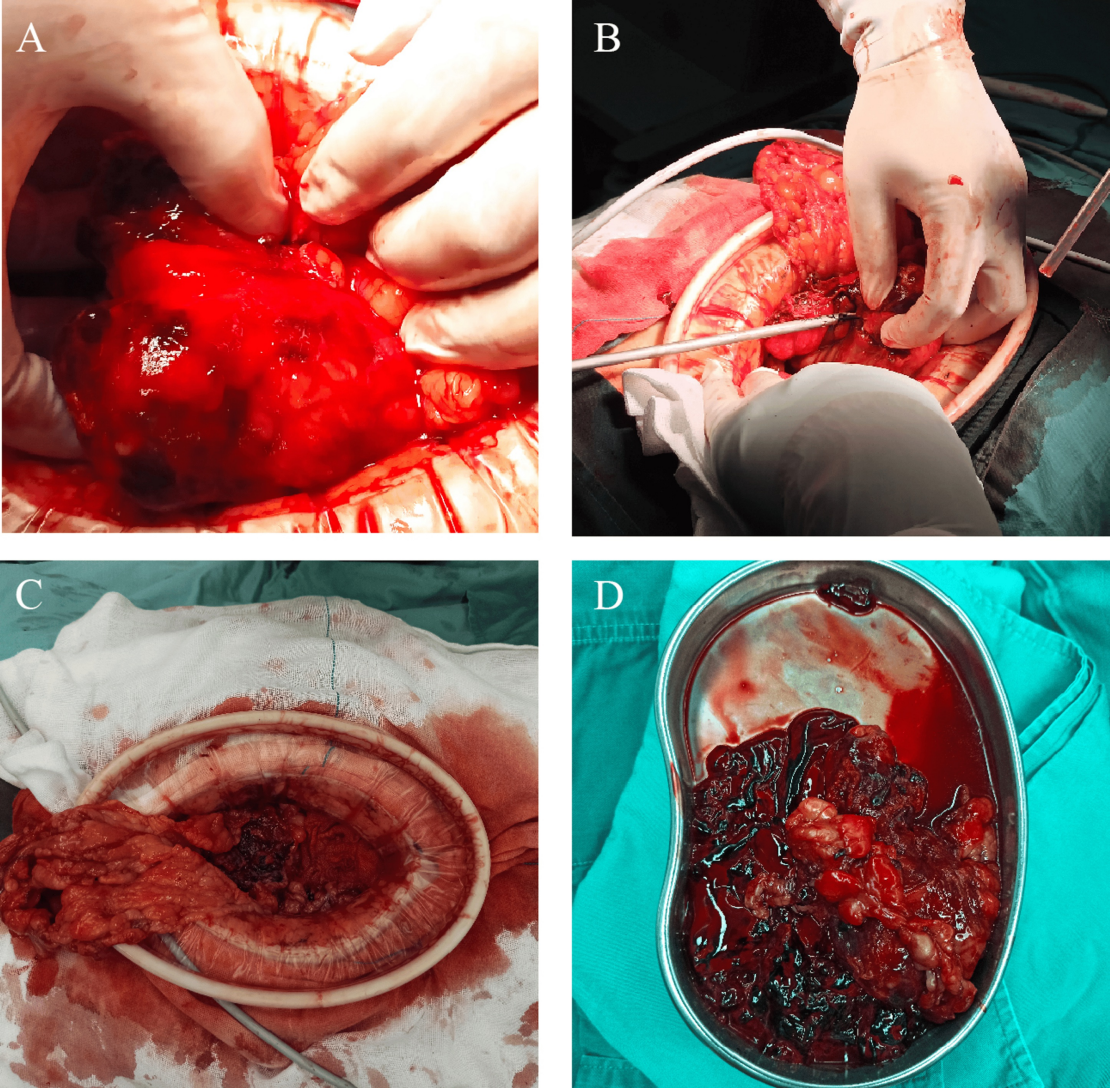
Intraoperative findings Revealed a tumor measuring 3.5 cm × 3 cm between the omental region and transverse colon (A, D). Resection of the tumor using an ultrasonic scalpel (B). No bleeding was observed at the surgical wound after the resection of the tumor (C).
